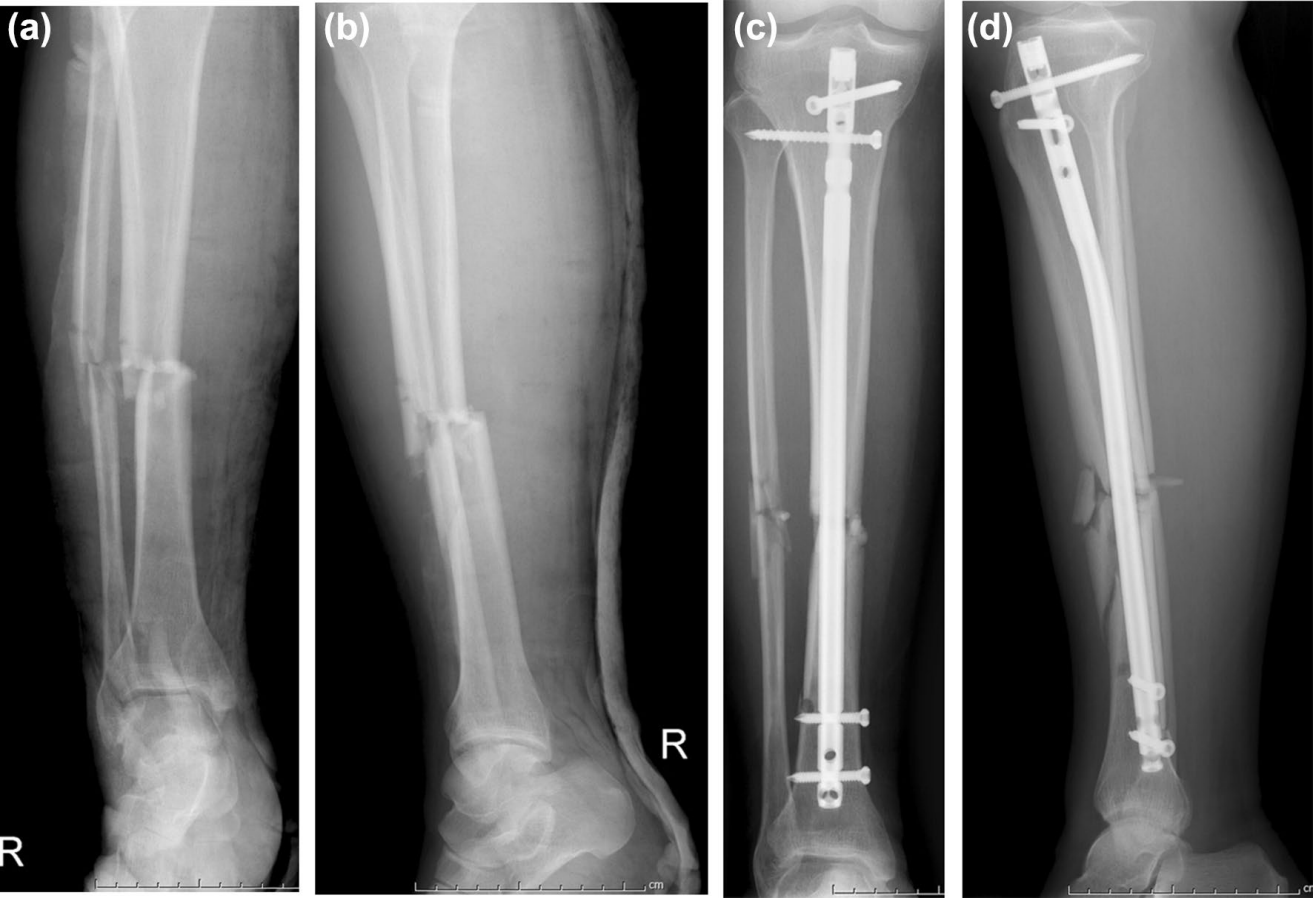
A 38-year-old male with the tibial shaft fracture. (a) The antero-posterior X-ray before surgery (b) the lateral X-ray before surgery, (c) the antero-posterior X-ray after surgery, (d) the lateral X-ray after surgery.
